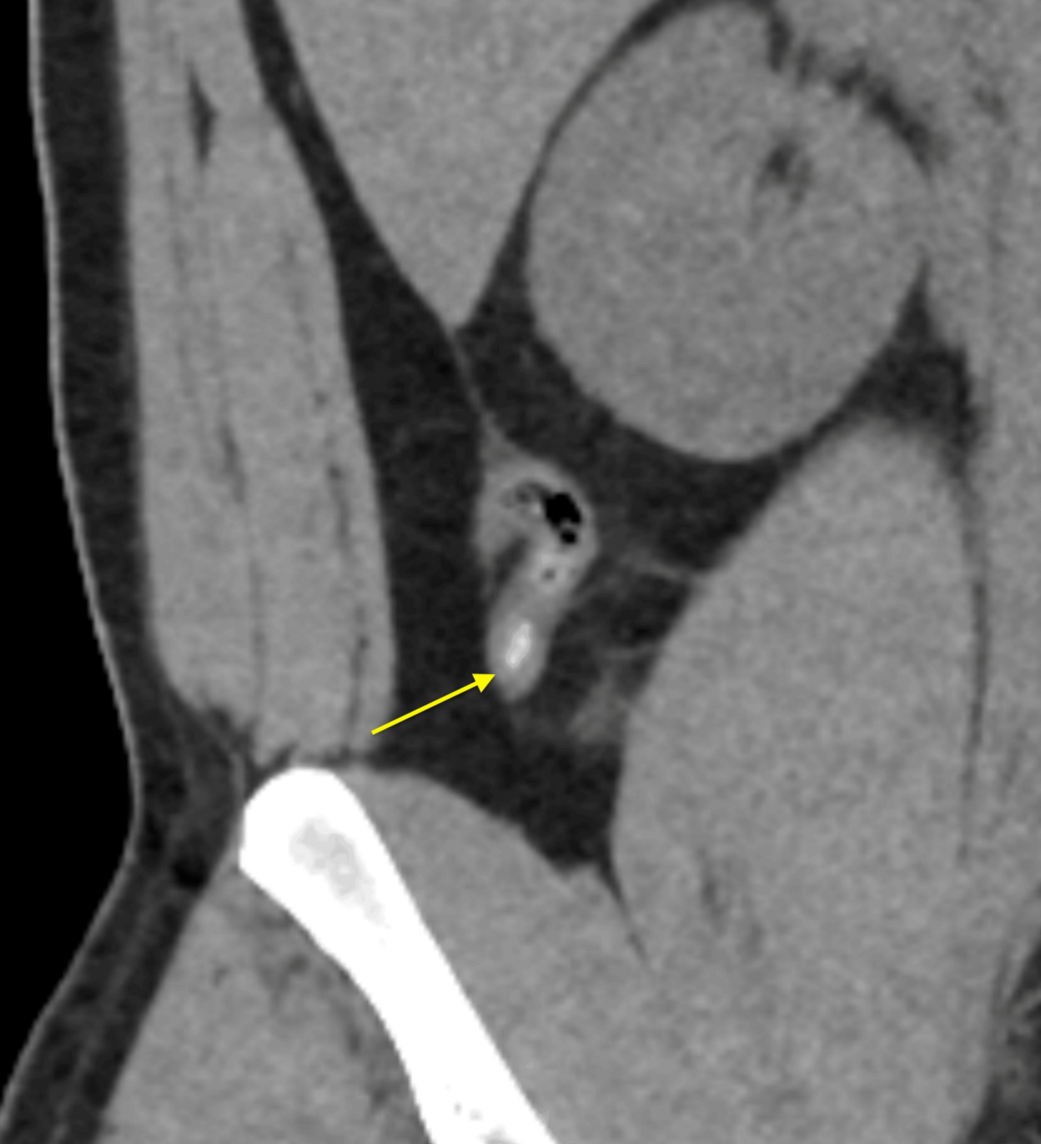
CT abdomen/pelvis without contrast showing a mildly thickened appendix measuring 8 mm without signs of perforation (yellow arrow).
